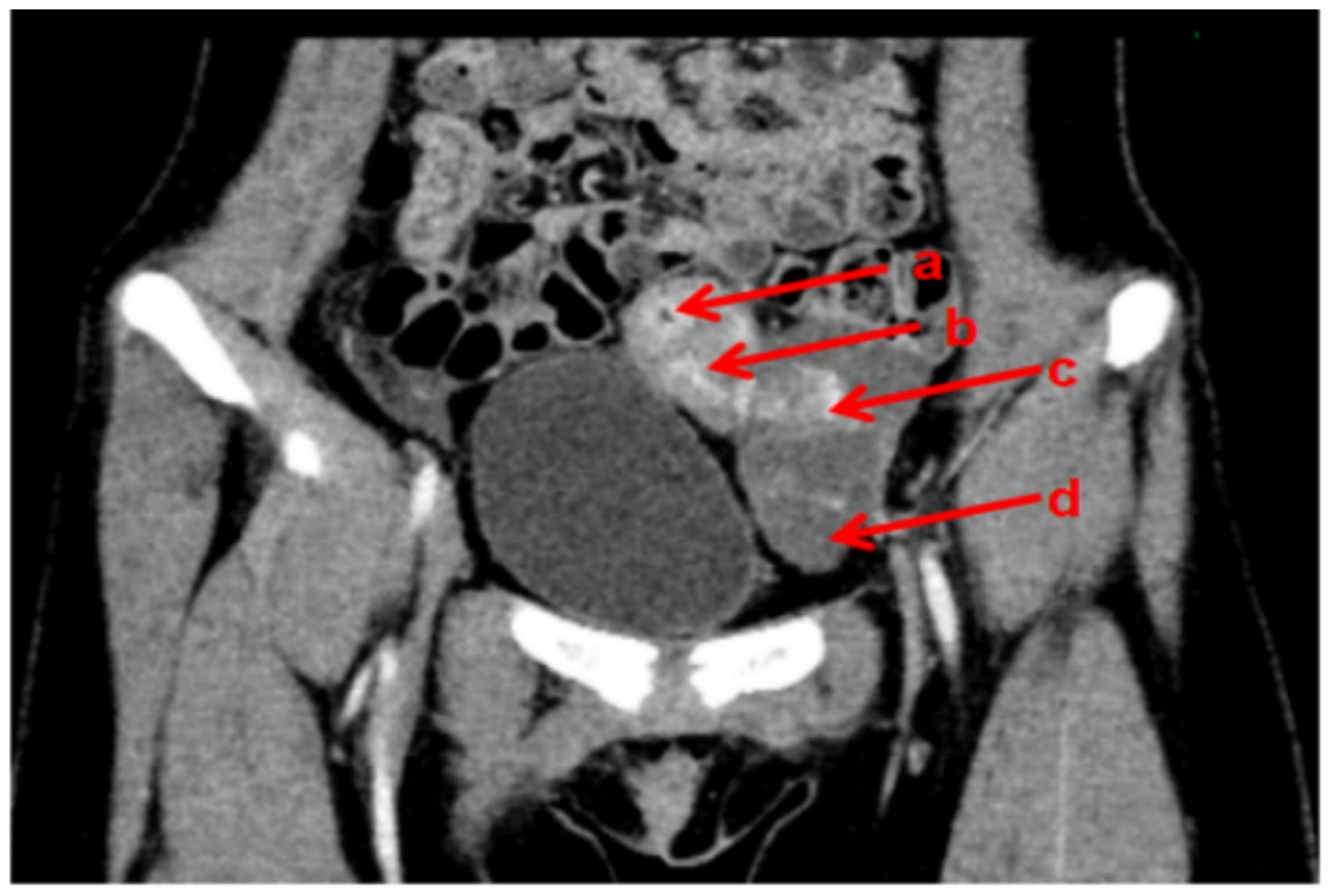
Preoperative CT images of the patient (a: uterine cavity; b: left rudimentary horn uterus; c: left ovary; d: left fallopian tube).
